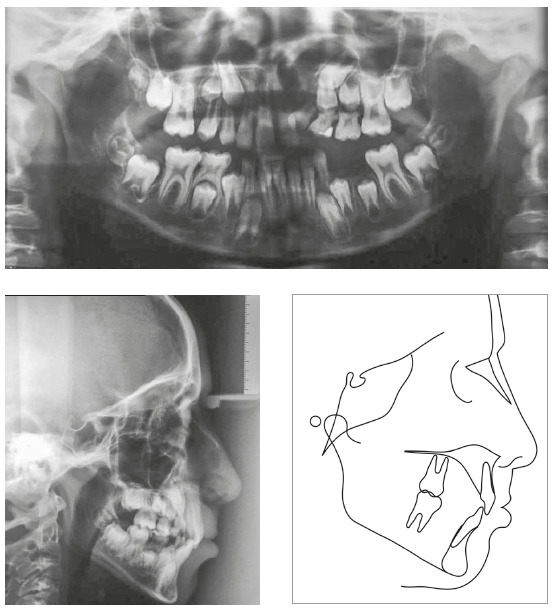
Initial radiographies and cephalometric tracing.
